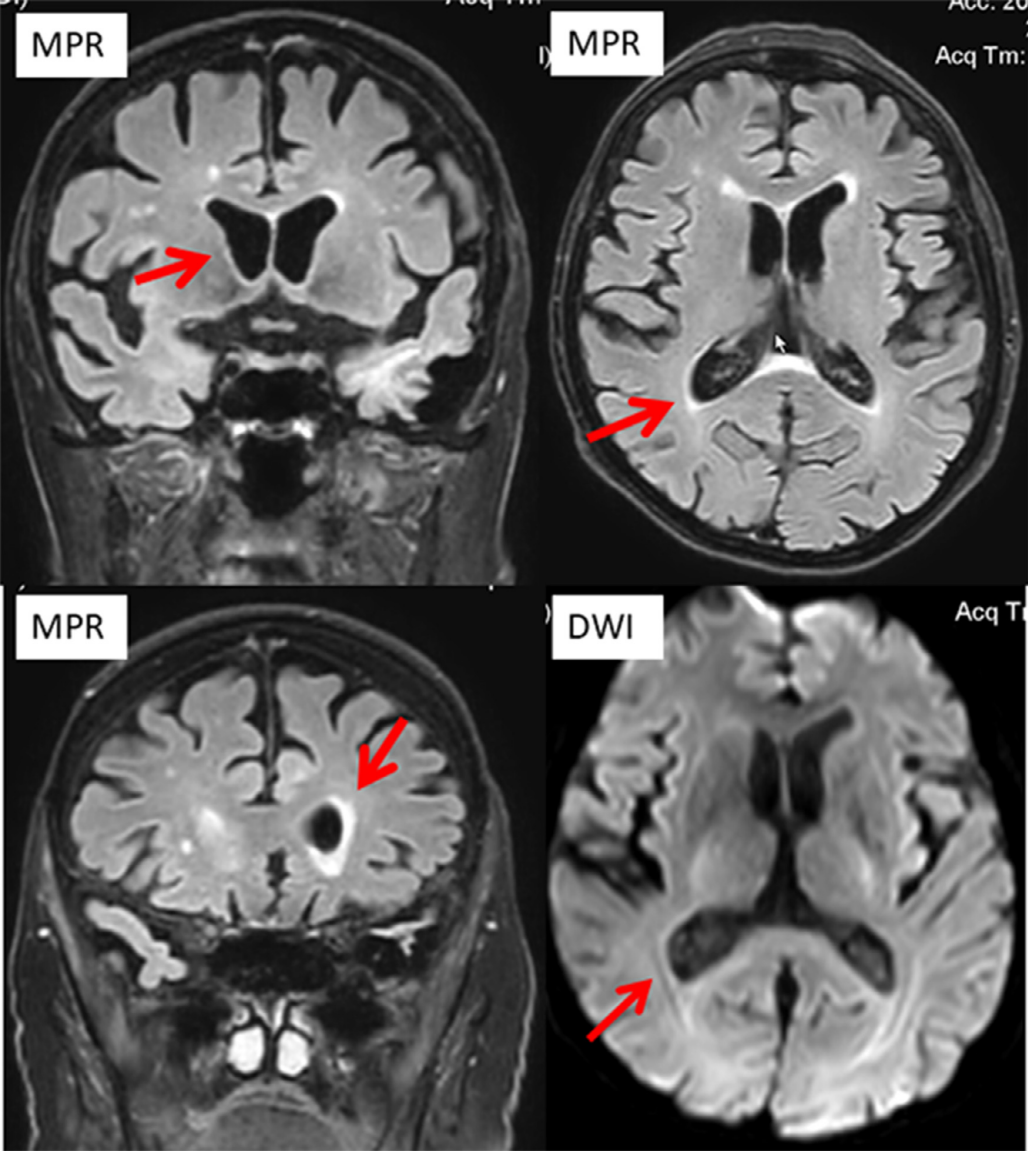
Cerebral MRI with contrast medium on hospital day 2 showing ependymal enhancement.
